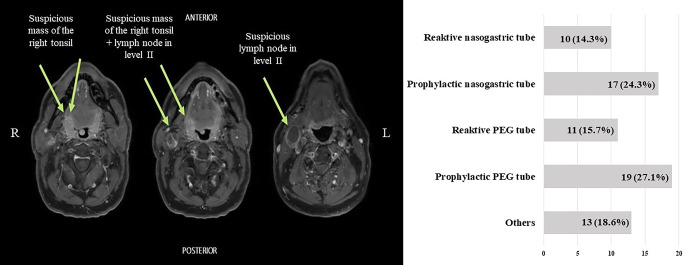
Participants’ answers on case report no. 3. The x‑axis represents the number of responses given. *PEG* percutaneous endoscopic gastrostomy, *MRI* magnetic resonance imaging, *BMI* body mass index, *ENT* ear, nose, throat
